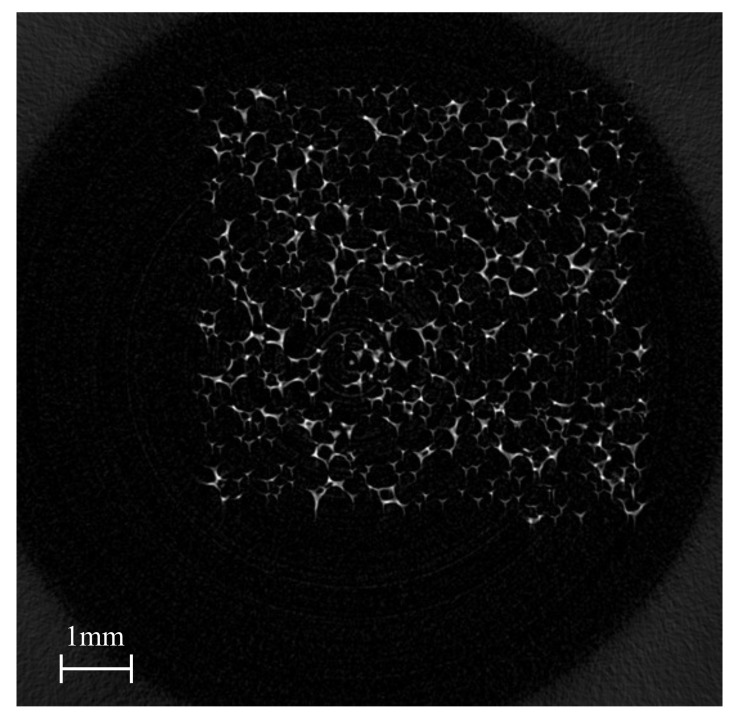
An example of the X-ray computed tomography (CT) scanned images for the polyurethane foams.
